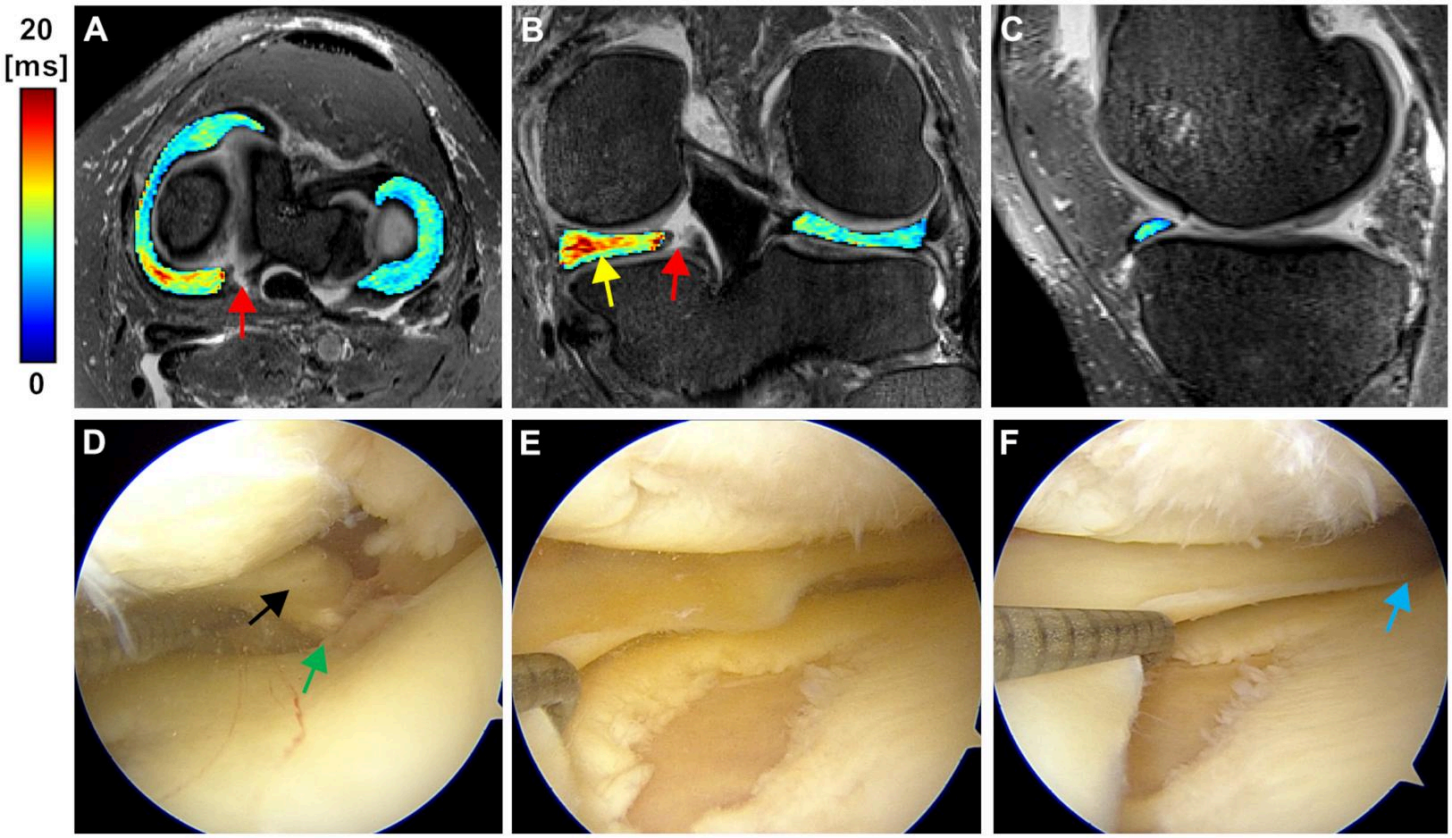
Representative MRI and arthroscopy images of a patient (age 57, female) with no arthroscopic findings (grade 0). A-C) 3D T2* maps overlaid on 3D SPACE fat-suppressed images of the medial and the lateral menisci with posterior horn root tear (arrow in A) in the medial meniscus. The segmented T2* maps show increased T2* values (arrow in B pointing to the colormap), particularly in the posterior region of the medial meniscus. The colour bar depicts T2* relaxation in milliseconds. D, E) Pre-operative arthroscopy images of the patient showing the medial meniscus root and the root attachment (arrows in D), and no signs of fibrillation of the medial meniscus. F) Post-operative arthroscopy image of the patient depicting the root repair (arrow).
